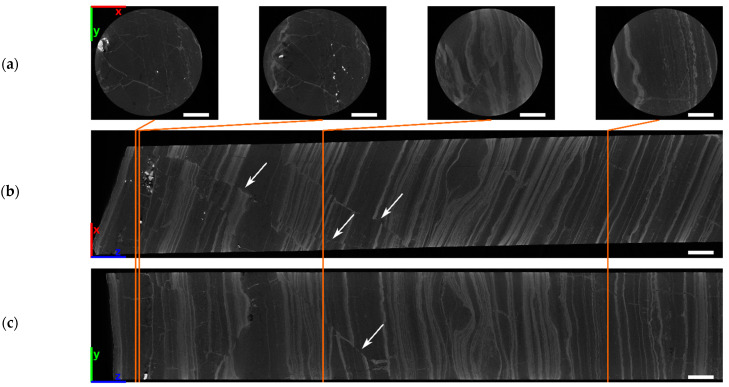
Virtual cross sections along different directions through the reconstructed CT volume of the banded iron drill core: (a) XY-plane; (b) XZ-plane; (c) YZ-plane. Highly absorbing inclusions (bright spots) are visible at the left end of (b) and (c). The left part also reveals microfaults (white arrows). Scale bars are 10 mm.
